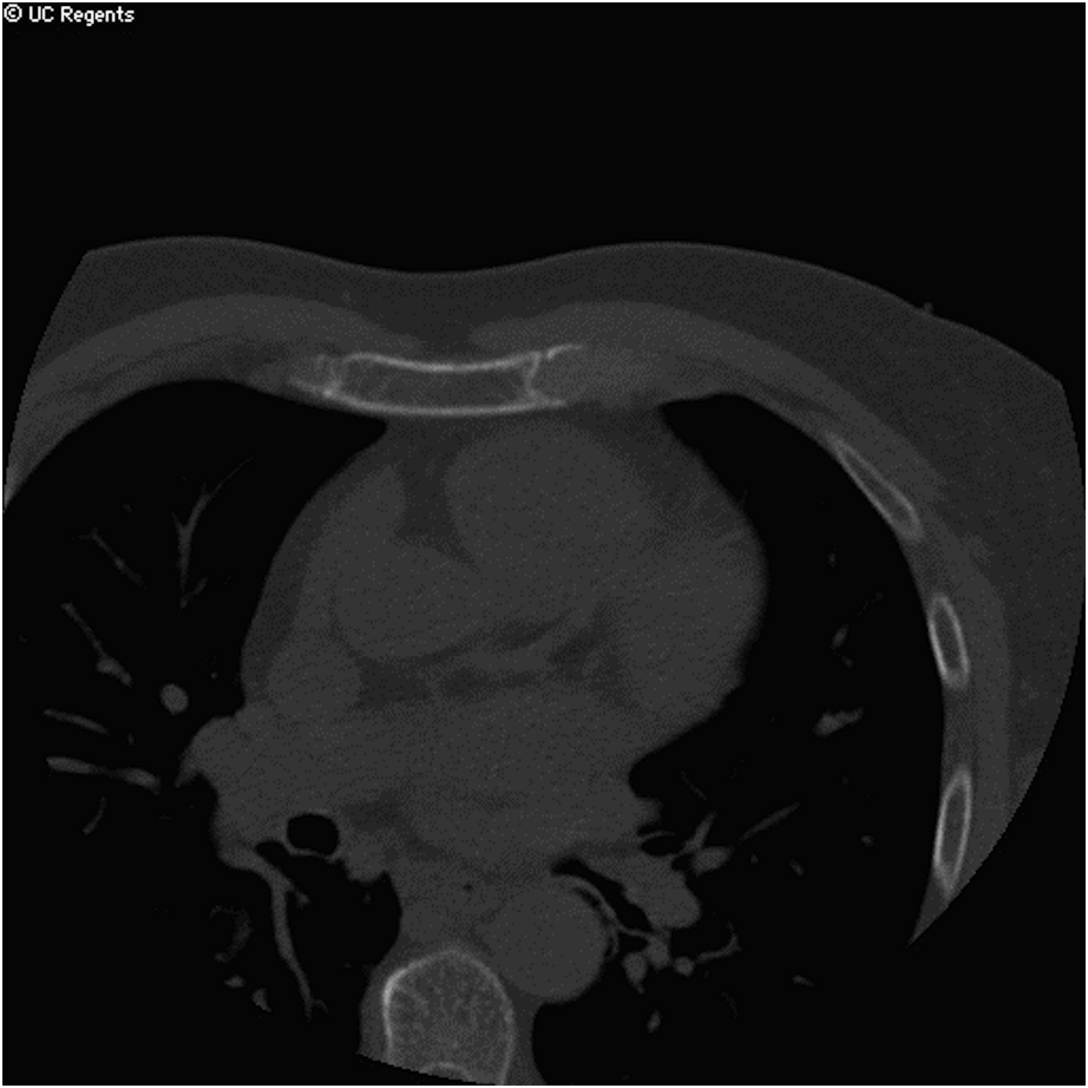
CAC EBCT of a subject with CAC score of 0. Abbreviations: Coronary artery calcium (CAC), External beam computed tomography (EBCT).
